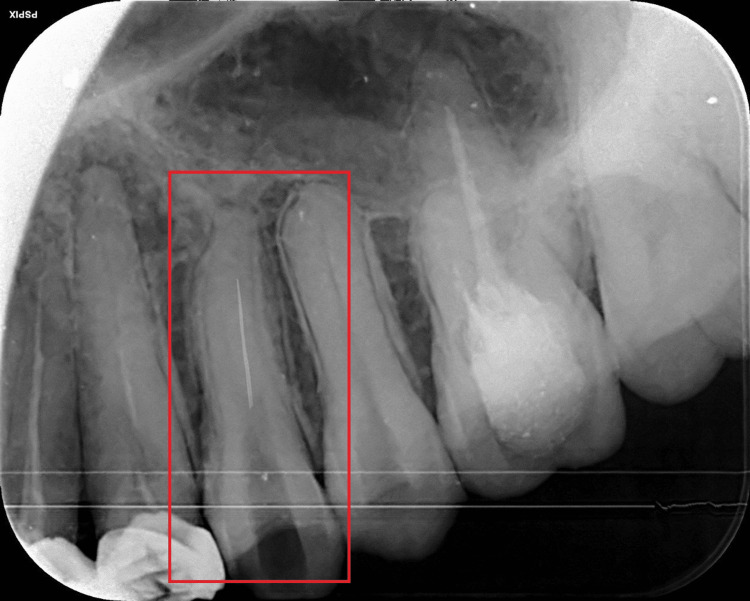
Radiographic confirmation of the separated instrument in 24 Preoperative radiograph of the separated instrument in 24
